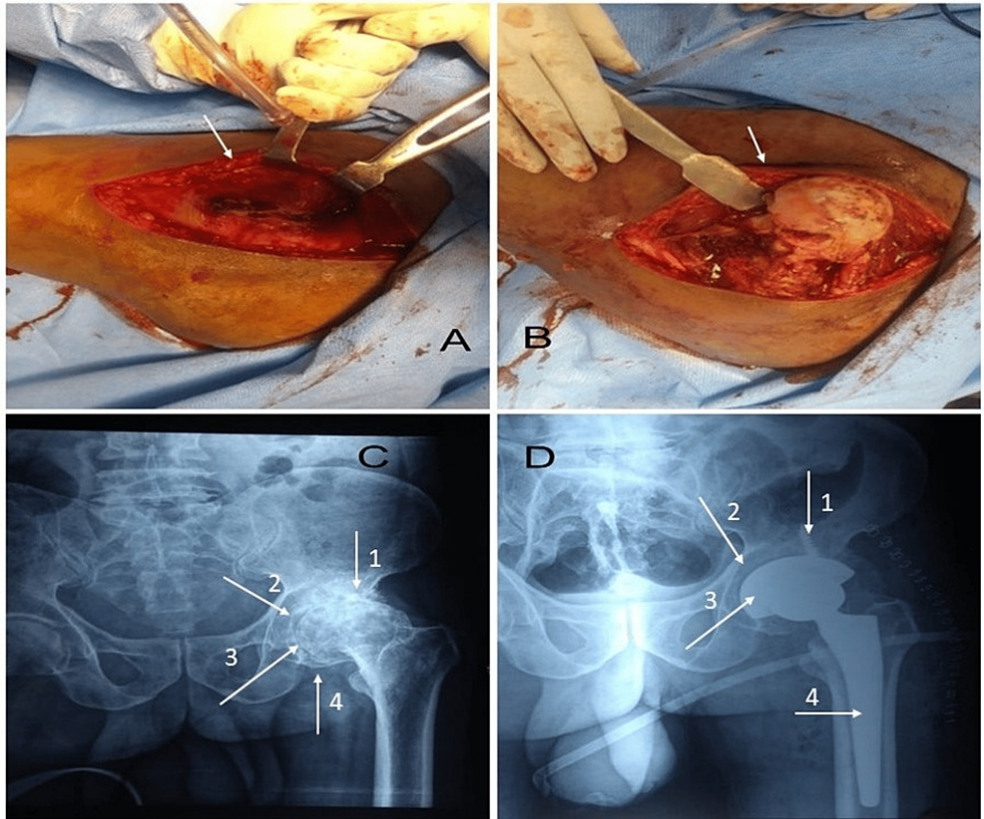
The lateral Hardinge approach A: the arrow shows the fascia split between the tensor fasciae latae (TFL) and gluteus maximus. B: the arrow shows the splitting of gluteus medius fibers at the anterior third and posterior two-third junction. C: radiographic image; arrow 1 shows subchondral sclerosis (looks more white ); arrow 2 demonstrates reduced joint space with a deformed femoral head; arrow 3 shows subchondral cyst; and arrow 4 shows osteophyte in the femoral head. D: radiographic image; arrow 1 shows the acetabular screw; arrow 2 shows the acetabular metal shell; arrow 3 points to the prosthetic femoral head; and arrow 4 shows the femoral stem
